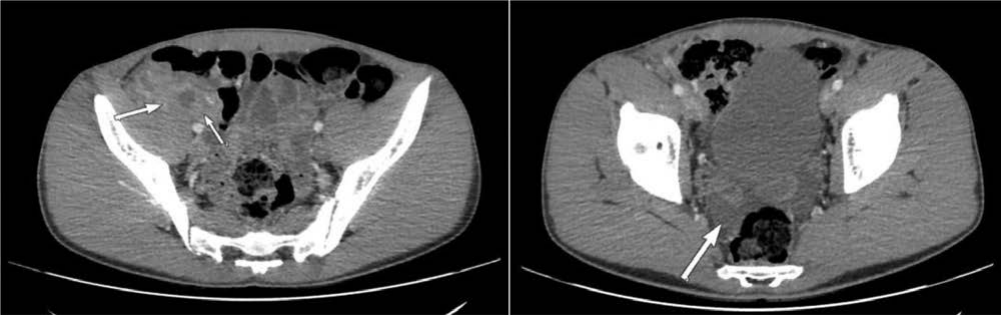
Abdomino-pelvic CT scan showing no significant abnormality of the last ileal loop. Several peri-centimeter lymph nodes were noted in the right iliac fossa, with a layer of fluid effusion. CT = computed tomography.
